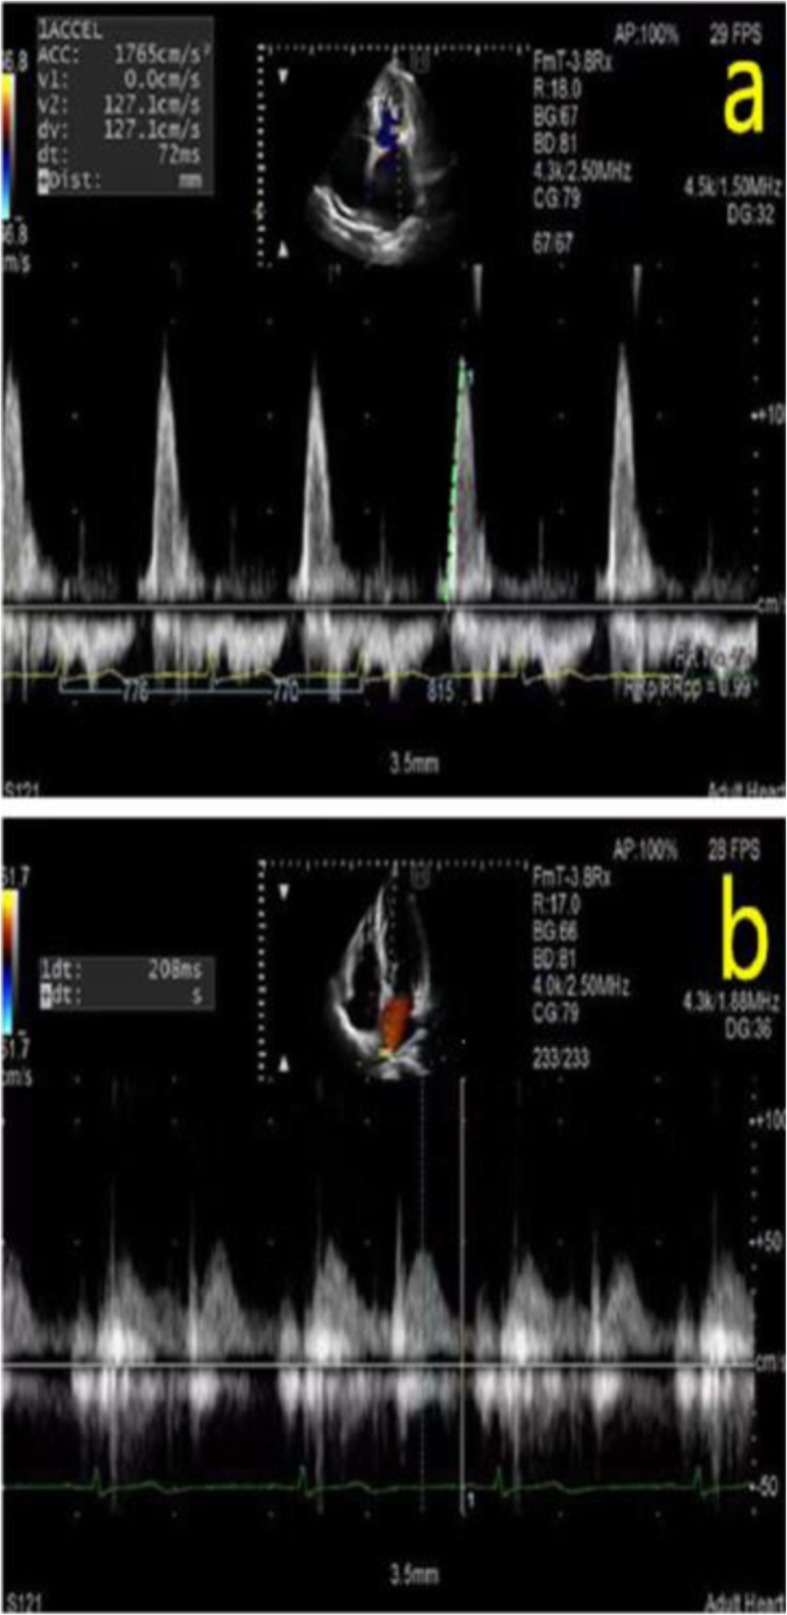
a Transmitral velocity was tracked and peak acceleration of the early transmitral flow peak velocity was measured. b. The flow of the right pulmonary vein was confirmed with the help of color doppler ultrasound in the apical four-chamber view and we measured deceleration time of pulmonary venous diastolic velocity
